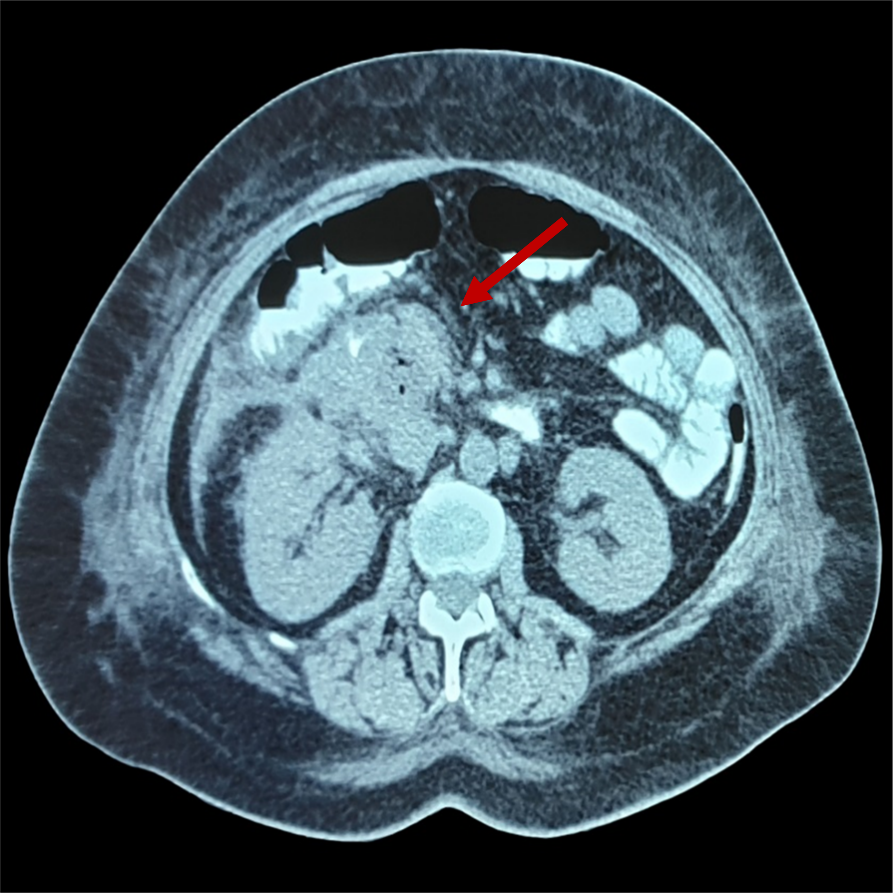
A CT scan of the abdomen shows a 5 cm abscess by the head of the pancreas
